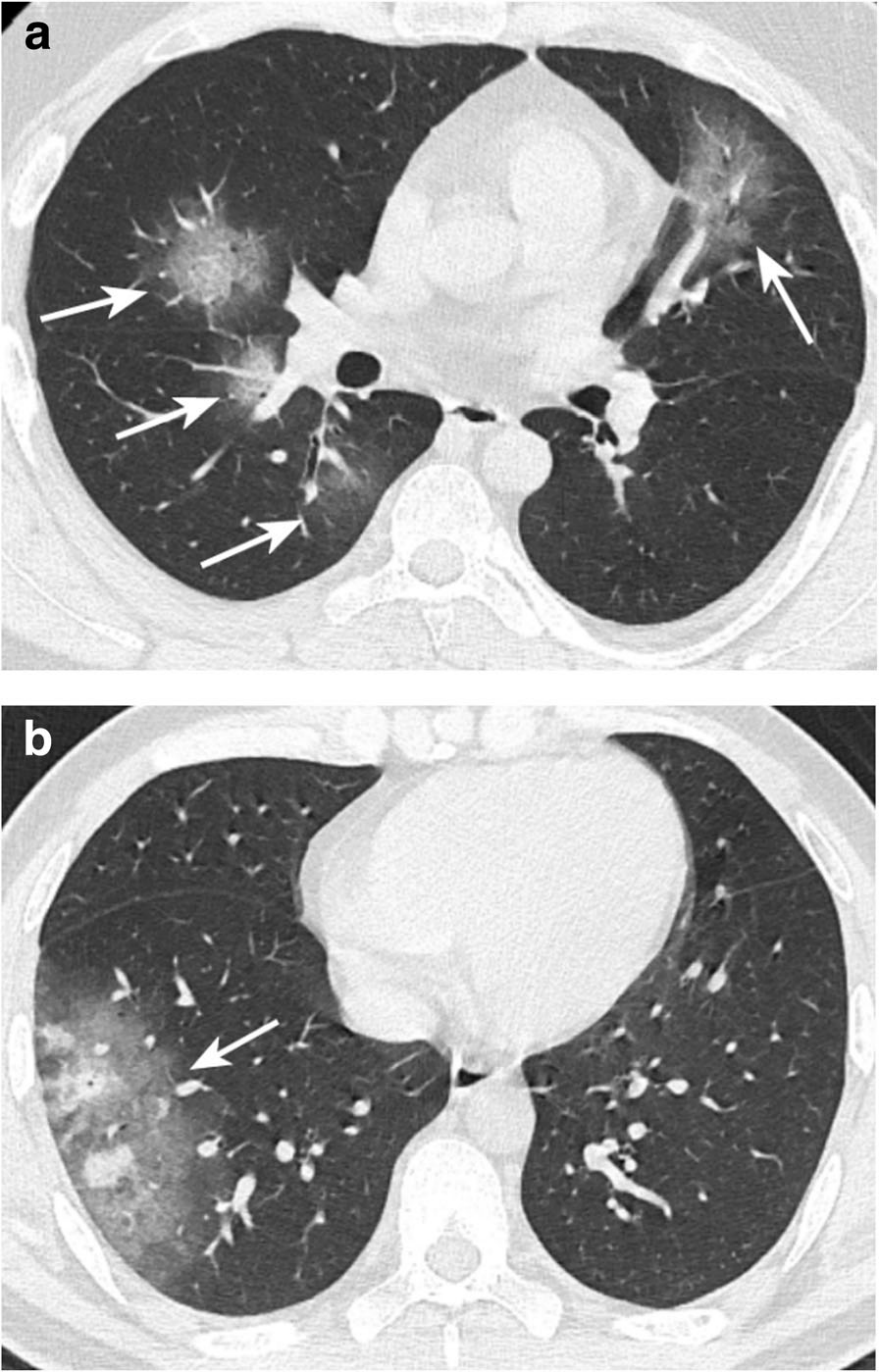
Typical pGGO case a: Moderate type patient. CT showed multiple pGGOs in the bilateral lung lobes with halo sign (arrow). Some of the lesions were located under the pleura. Typical mGGO case b: Moderate type patient. CT showed a subpleural mGGO within a solitary nodule (arrow)
